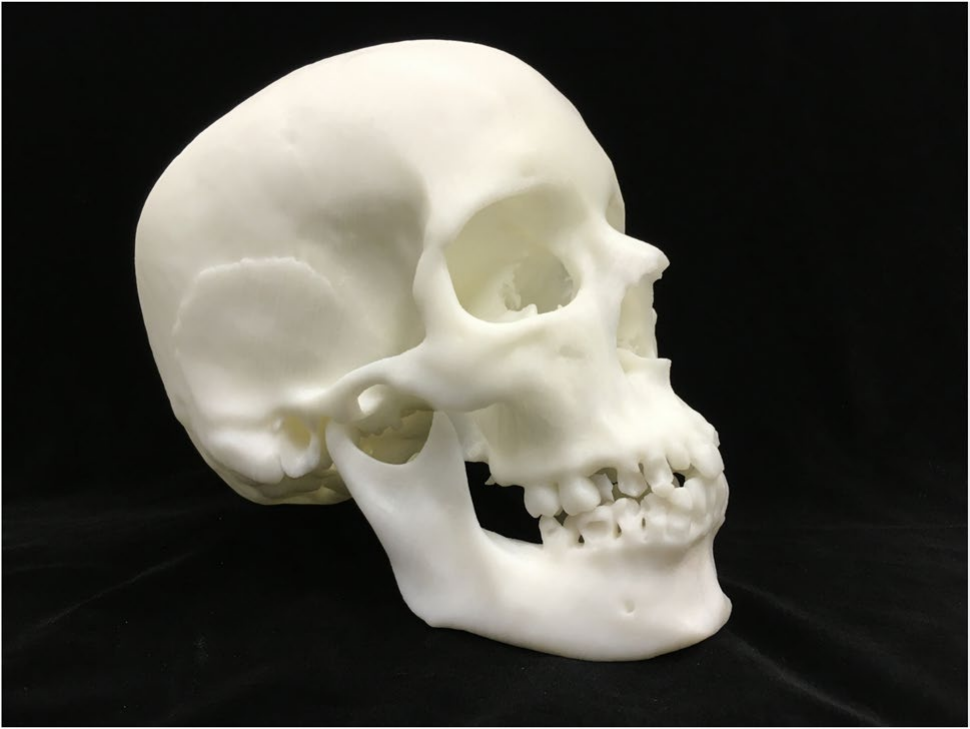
3D-printed skull extracted from a CT scan using FDM printing
